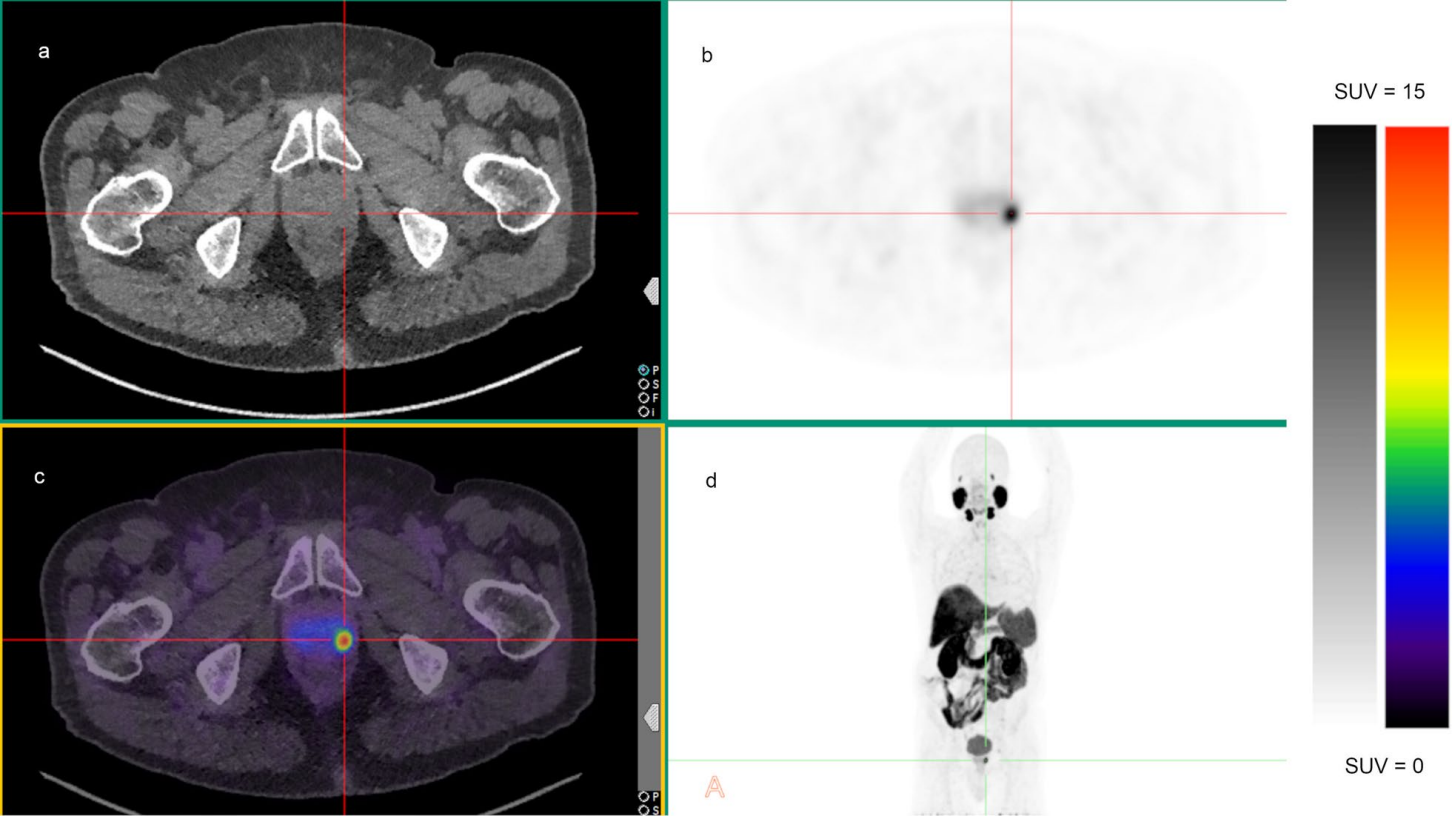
A 70-year-old male with unfavourable intermediate-risk prostate cancer (ISUP grade 3; PSA 5.2 ng/mL) who underwent [^18^F]PSMA-1007 PET/CT that showed a solitary F-PSMA avid focus within the left mid posterolateral prostate gland (SUVmax 14.9). a Axial non-contrast CT, b axial PET attenuation corrected image and c axial fused PET/CT image at the level of the prostate gland. d Maximum intensity projection (MIP) image
